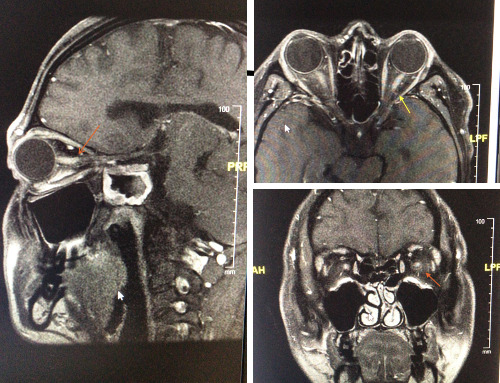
MRI: mild bilateral contrast enhancement on the contrast-enhanced images in the optic nerves’ retrobulbar segment (arrows)
